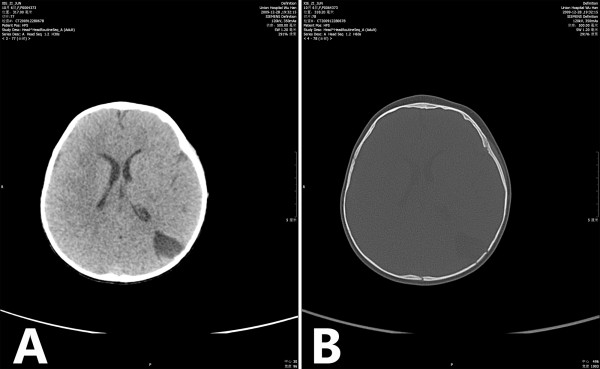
Brain CT. (A) 2.5 × 2.4 cm low density area in left parietal lobe; (B) the interrupted skull continuity in left parietal bone and left occipital.
